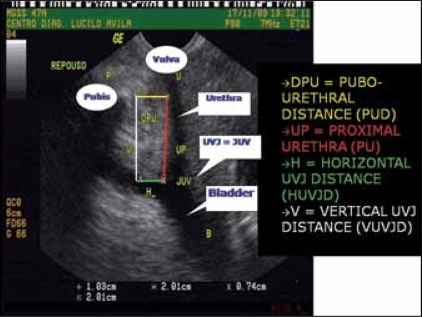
Transvulvar ultrasound image showing how the VUVJD, HUVJD, PUD and PU have been measured
